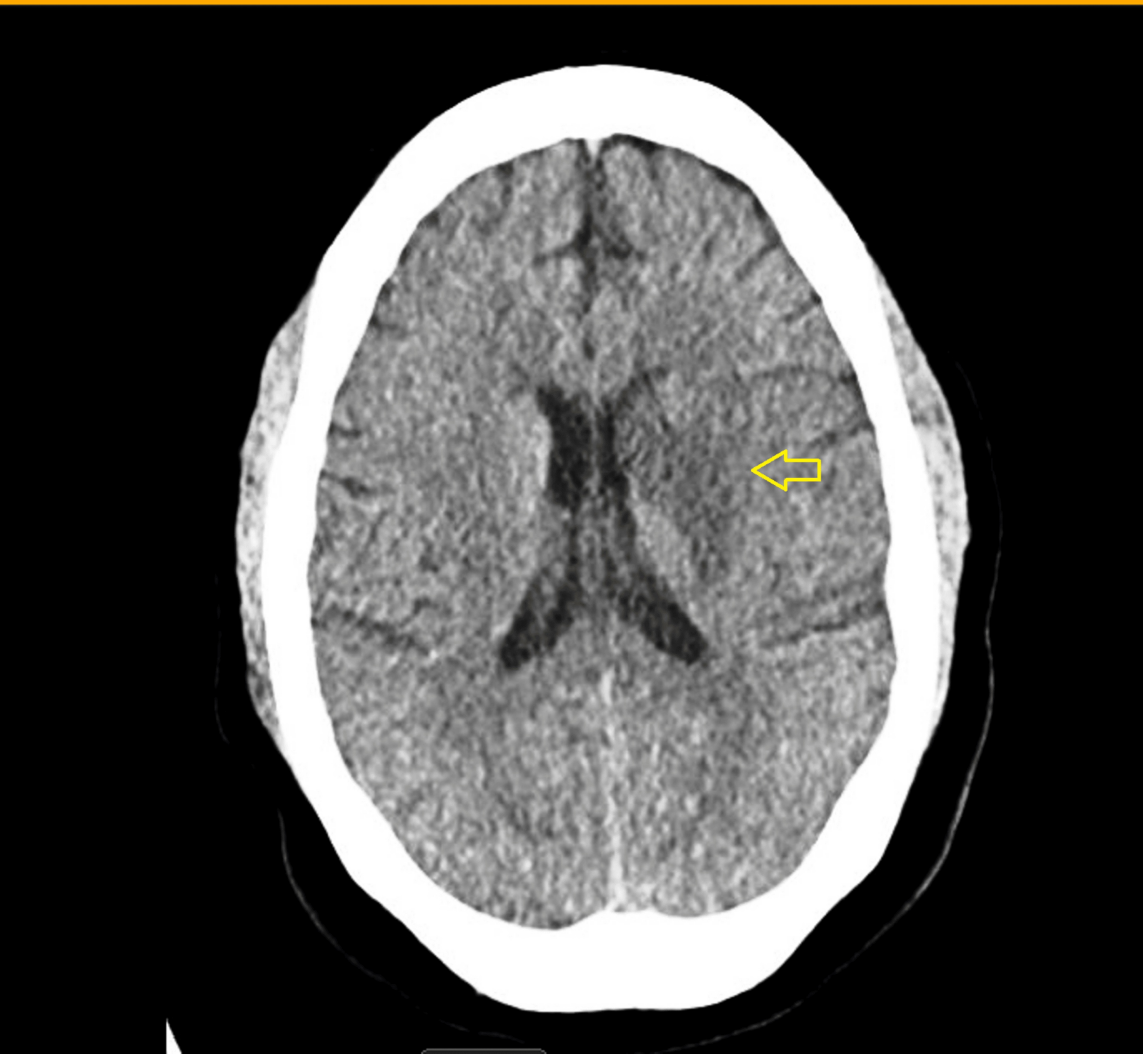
CT Head without contrast: Left frontal-parietal lobe infarct within the middle cerebral artery (MCA) territory (yellow arrow). This will lead to the right side hemiplegia, hemisensory loss, gaze deviation and neglect syndrome.
